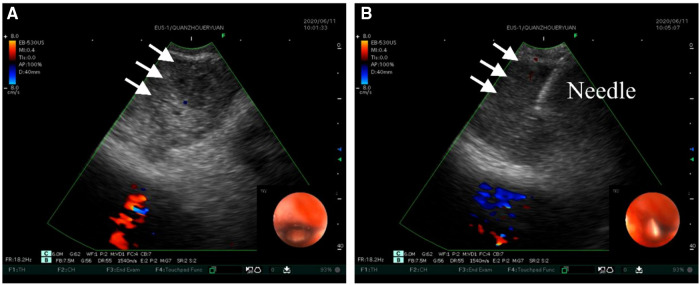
Ultrasound images of EBUS-TBNA. (A) Convex-probe ultrasound demonstrates the hypoechoic lesion (arrows), while the endoscopic Doppler image reflects the blood flow within the right atrium. (B) EBUS-TBNA was performed using a 22-gauge needle.
